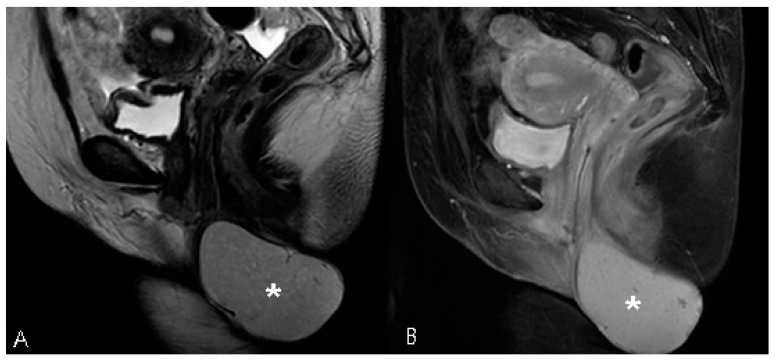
Magnetic resonance image showing a well-defined perineal mass (asterisk) with (A) intermediate signal intensity on the T1-weighted image and (B) intermediate signal intensity on the T2-weighted image.
